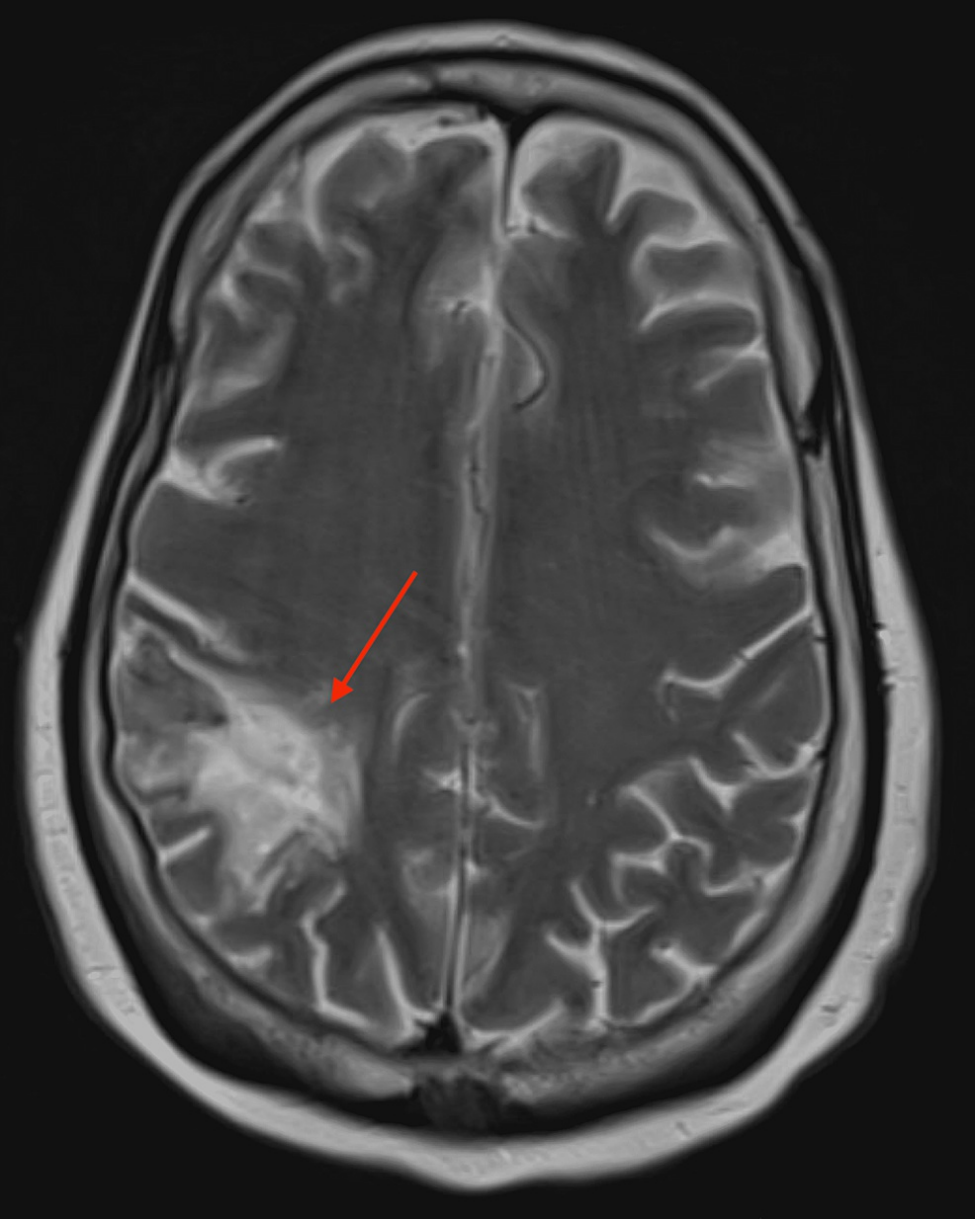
MRI of the brain - image 1 The red arrow is pointing at the chronic right parietal lobe lesion in this T2-weighted MRI image MRI: magnetic resonance imaging
